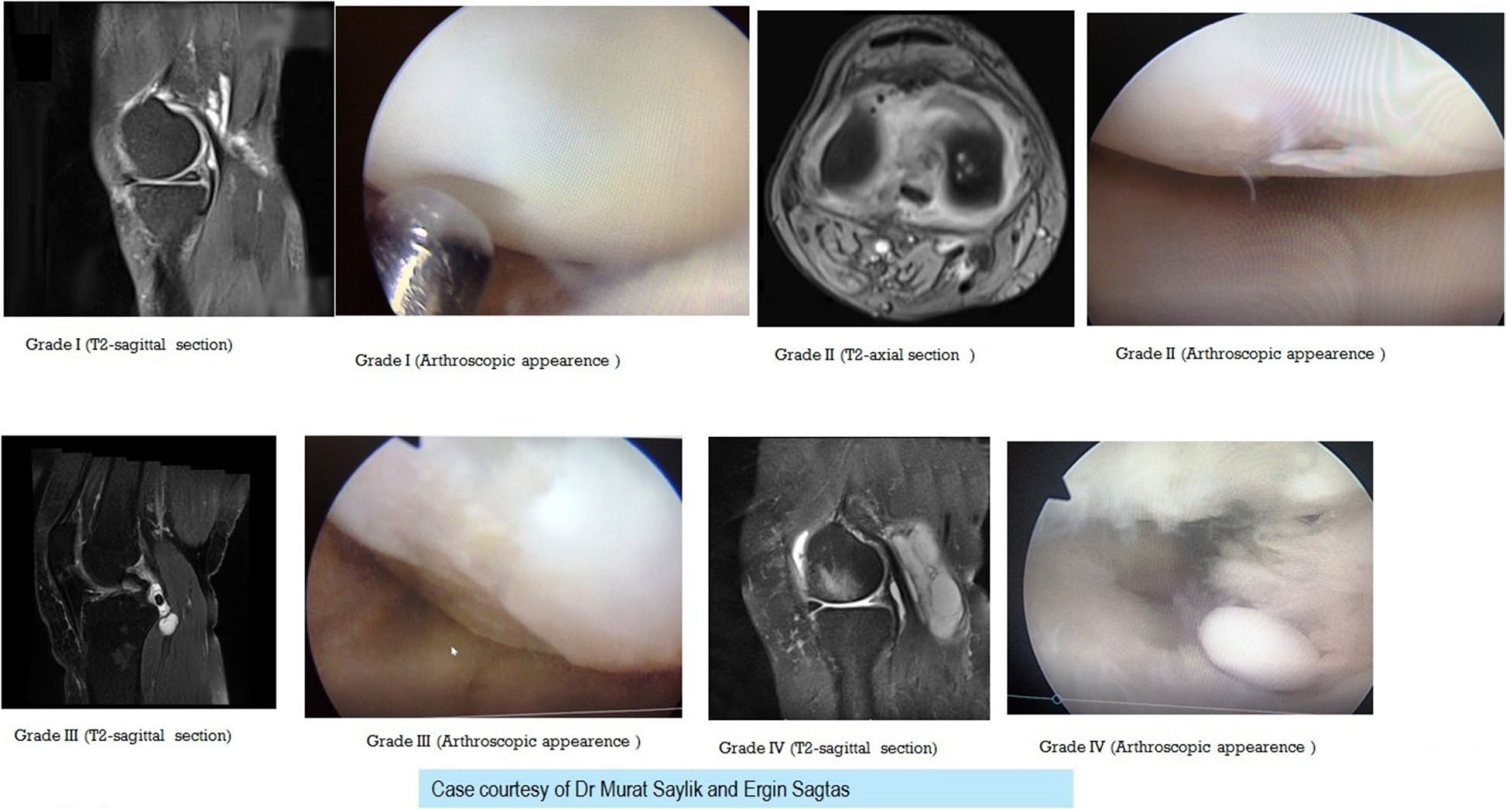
Depicts the grade 1,2,3,4 chondral lesions according to the the outerbridge classification on MRI and arthroscopic images together
